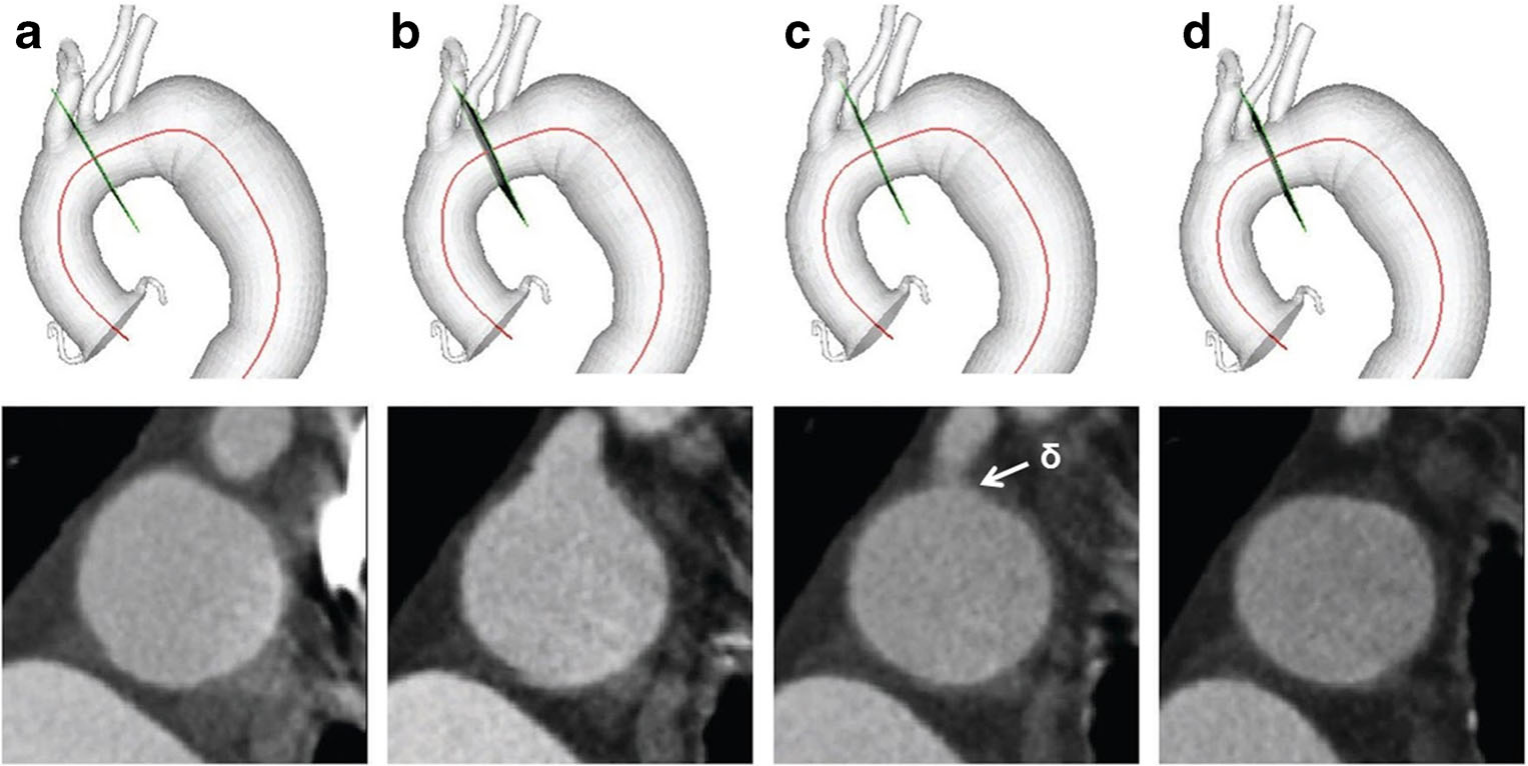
Location of the Greenwich point *δ* on a CT-based anatomic model. Orthogonal cross-sections to the mother vessel centerline a completely proximal to the daughter vessel where the daughter vessel is not visible, b at the daughter vessel where the daughter vessel appears as a “bud,” c at the most distal intersection of the mother and daughter vessels where the intersection point is chosen as *δ*, and d completely distal to the daughter vessel where the mother and daughter vessels are separate
